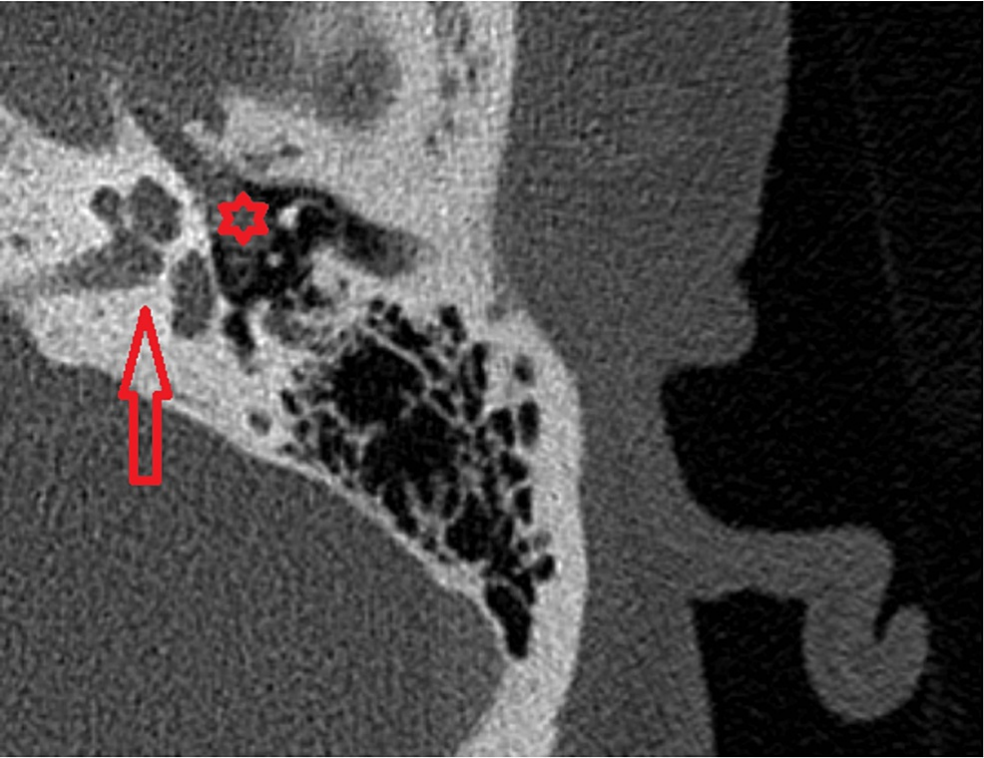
High-resolution axial CT IAC without contrast showing the left middle ear filled with soft tissue involving the incudostapedial joint CT: computed tomography; IAC: internal auditory canal
